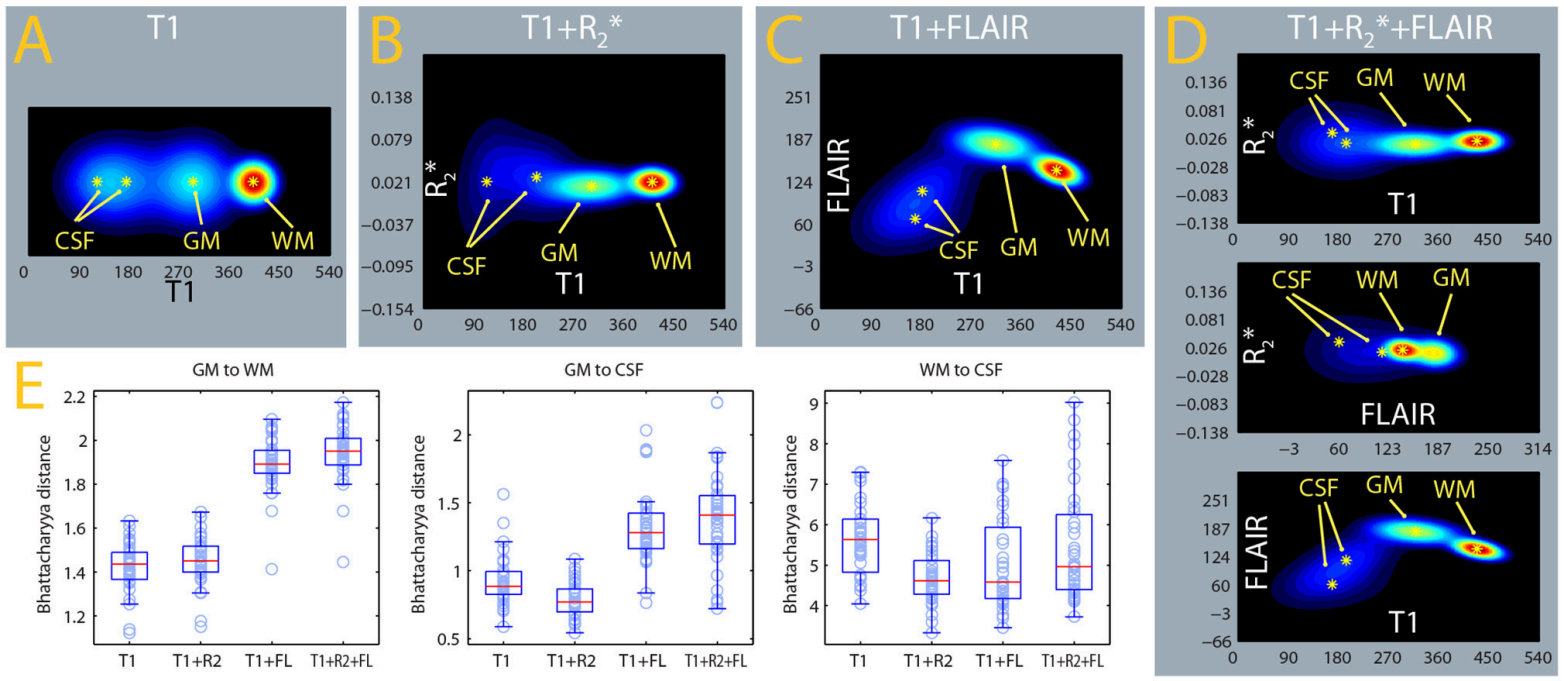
Estimates of tissue class densities and relative separation. (A–D) : average signal densities from the MPRAGE, MPRAGE+${\text{R}}_{2}^{*}$, MPRAGE+FLAIR, and MPRAGE+${\text{R}}_{2}^{*}+$FLAIR segmentations. The estimate from the MPRAGE segmentation alone was displayed as a two-dimensional density to facilitate comparison. The gray matter (GM) and white matter (WM) tissue classes were modeled with one Gaussian, while cerebrospinal fluid (CSF) was modeled as a mixture of 2 Gaussians. (E) Box-plots of the Bhattacharyya distances between the tissue classes computed in the individual segmentations. The distance of GM to CSF (box-plot in the middle) was computed to the Gaussian with largest weight in the mixture.
